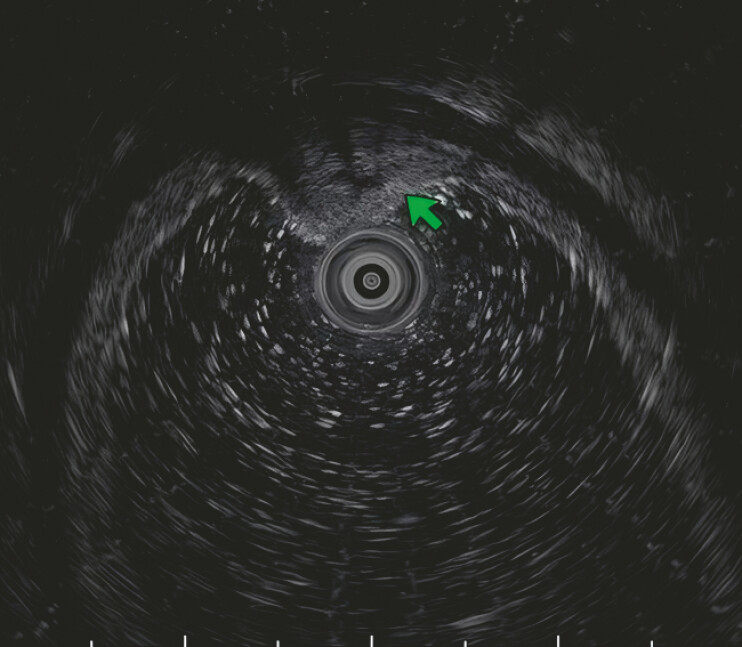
Endoscopic ultrasonography revealed a heterogeneous medium-to-high echogenicity with anechoic structures visible internally, originating from the submucosal layer.
